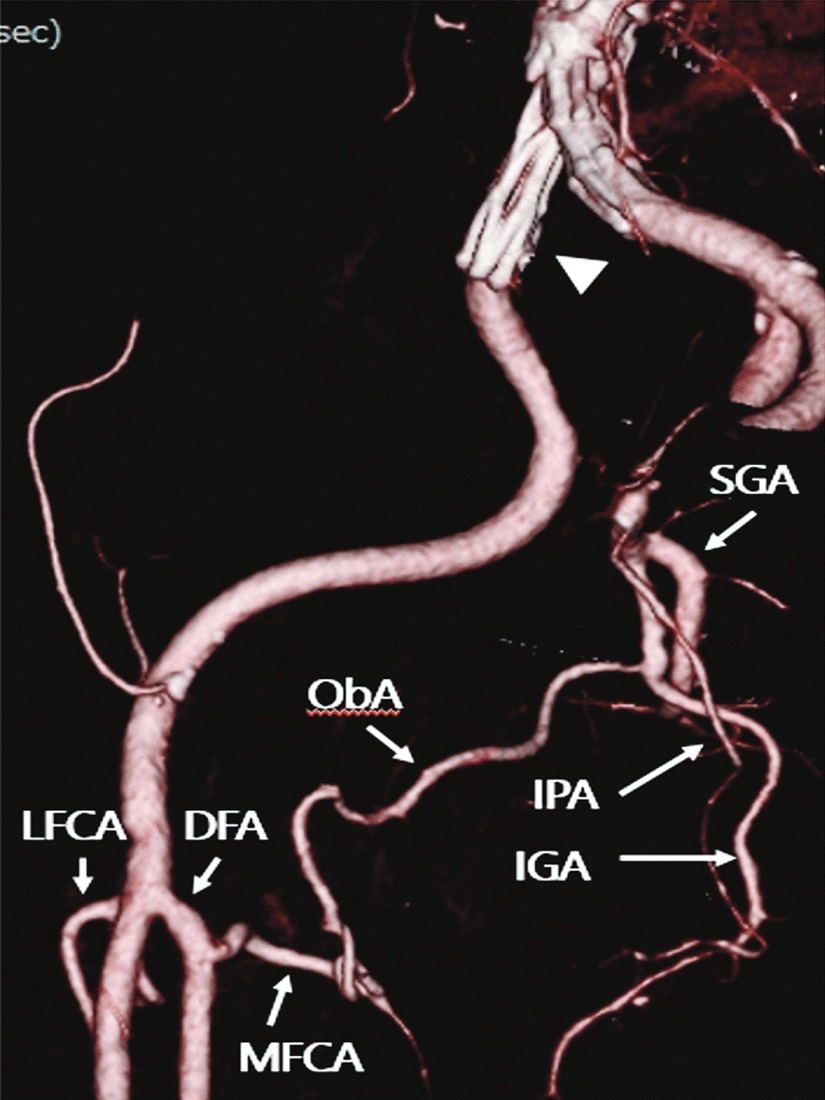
3DCTA after endovascular aneurysm repair with internal iliac artery coverage (arrowhead). 3DCTA shows the ipsilateral MFCA-ObA pathway arising from the DFA which communicated with the IGA. *3DCTA* three-dimensional contrast-enhanced computed tomography angiography, *DFA* deep femoral artery, *LFCA* lateral femoral circumflex artery, *MFCA* medial femoral circumflex artery, *ObA* obturator artery, *SGA* superior gluteal artery, *IGA* inferior gluteal artery, *IPA* internal pudendal artery
